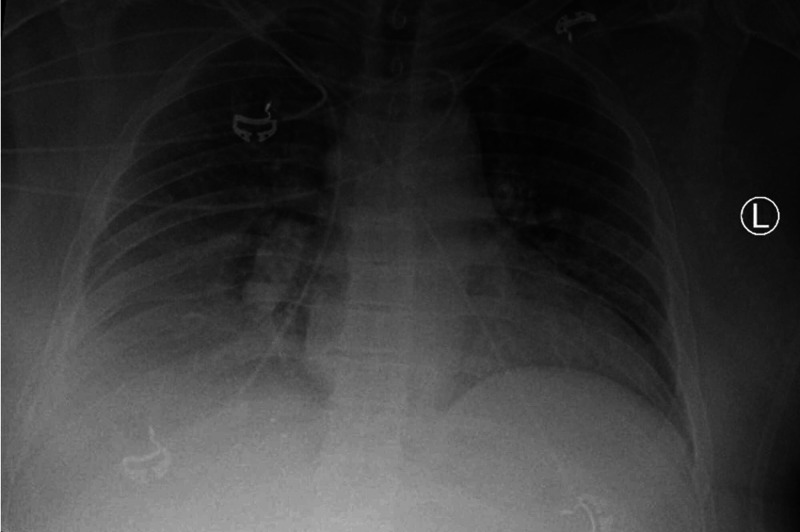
Repeat chest X-ray Anterior-posterior (AP) view, previously seen right pleural effusion has significantly decreased in size. Previously seen mediastinal shift has improved.
